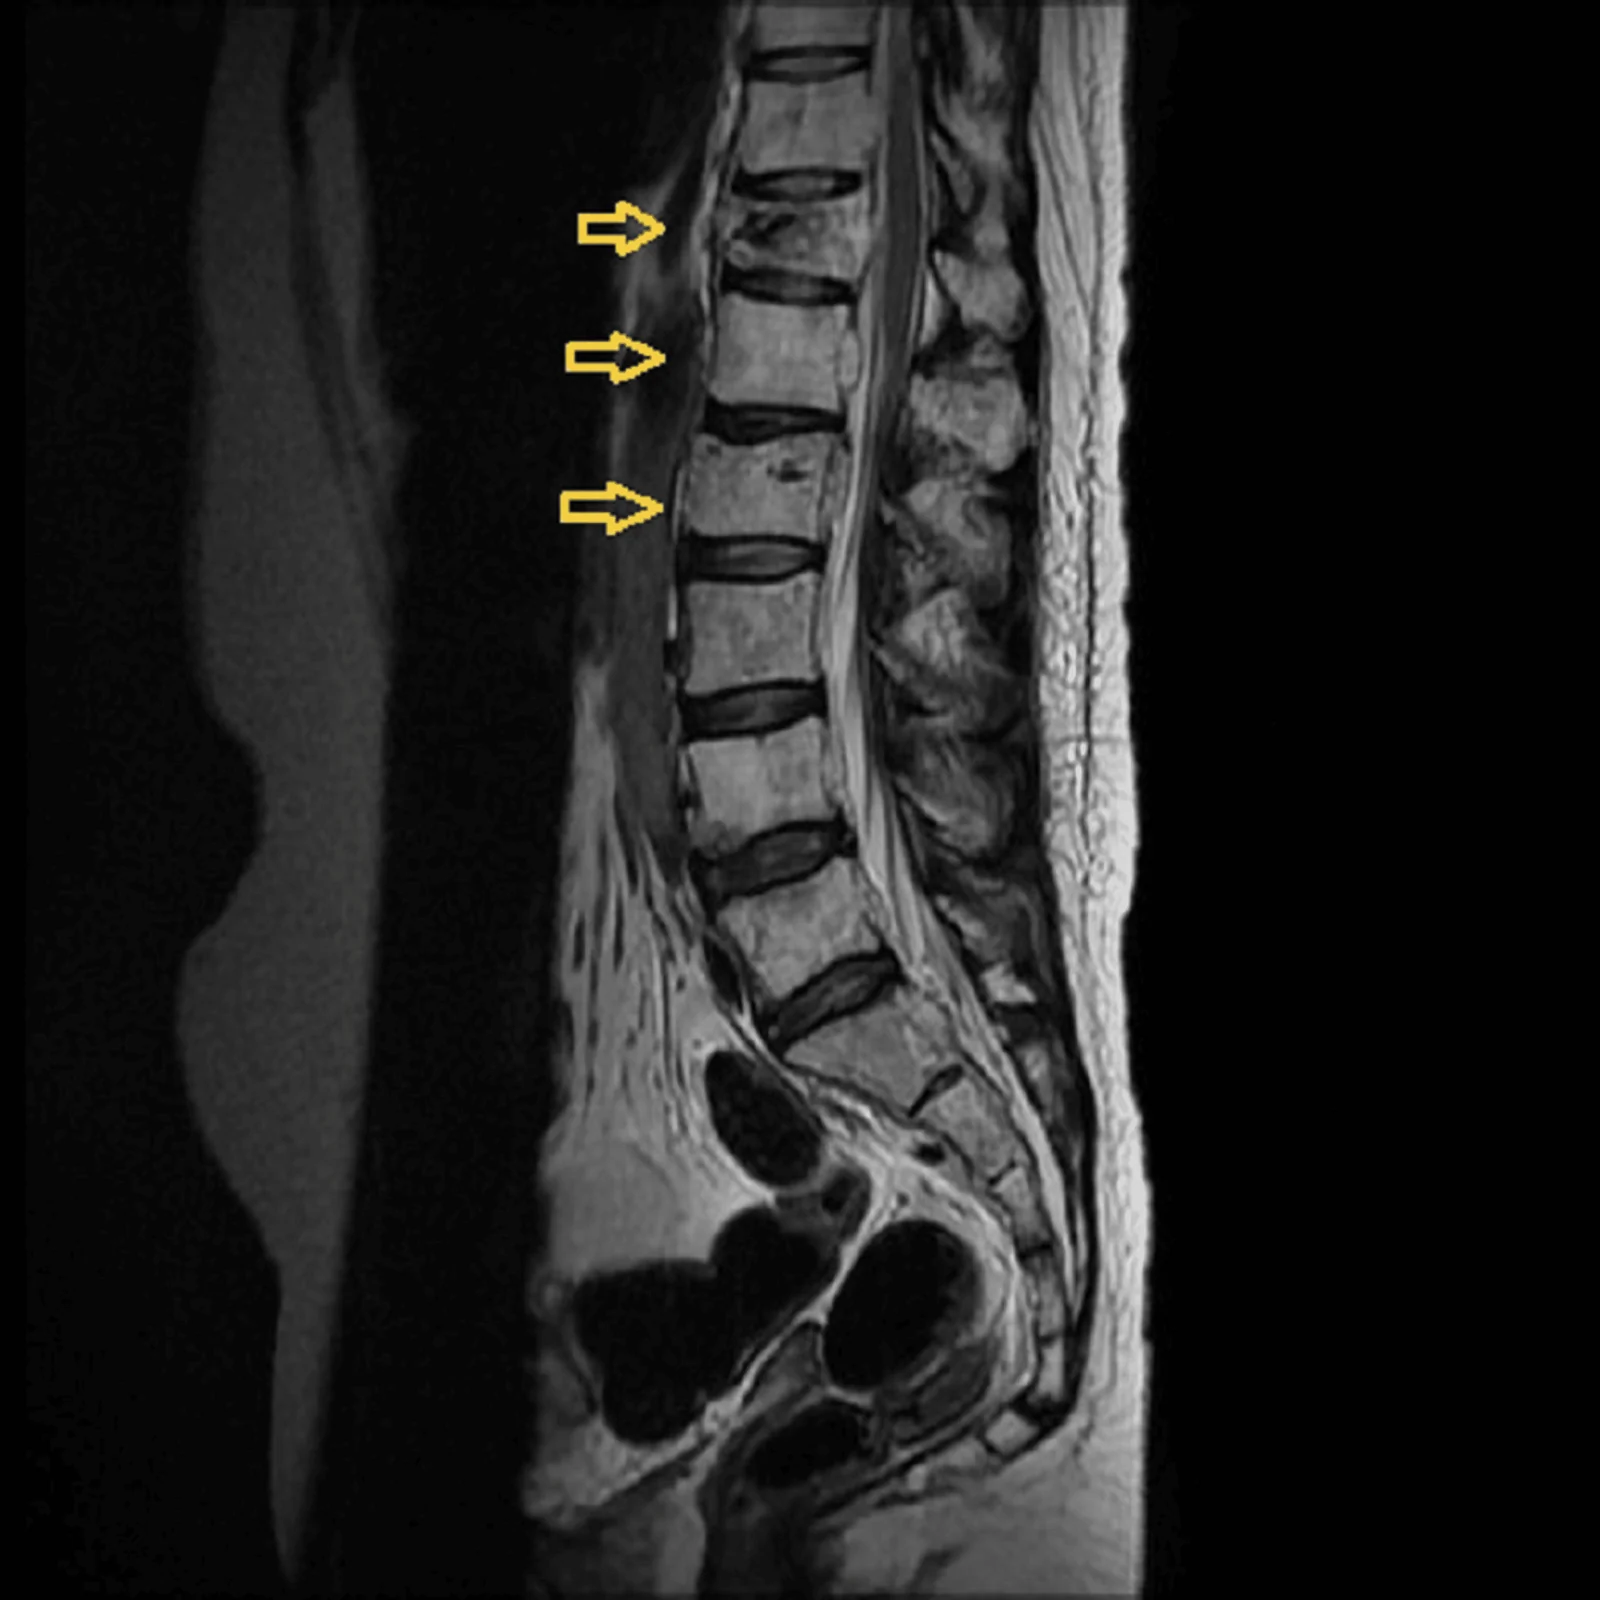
MRI of the spine post kyphoplasty showing a stable lumbar spine, with no retropulsion, facet subluxation, or segmental malalignment (arrows).
